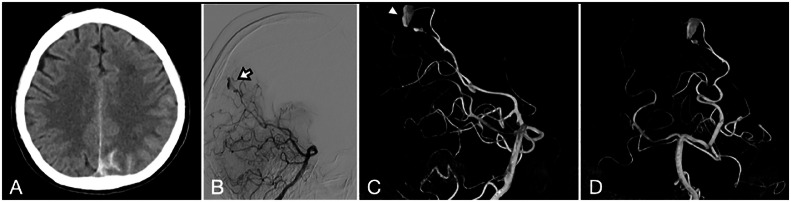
Axial non-contrast-enhanced CT head scan revealing subarachnoid haemorrhage in the left parieto-occipital region (A). Catheter angiogram after two-weeks of intravenous antibiotics demonstrating an enlarged left distal posterior cerebral artery aneurysm (B, white arrow, left vertebral artery injection lateral view; C, white arrowhead, AP view 3D reconstruction; D, lateral view 3D reconstruction).
